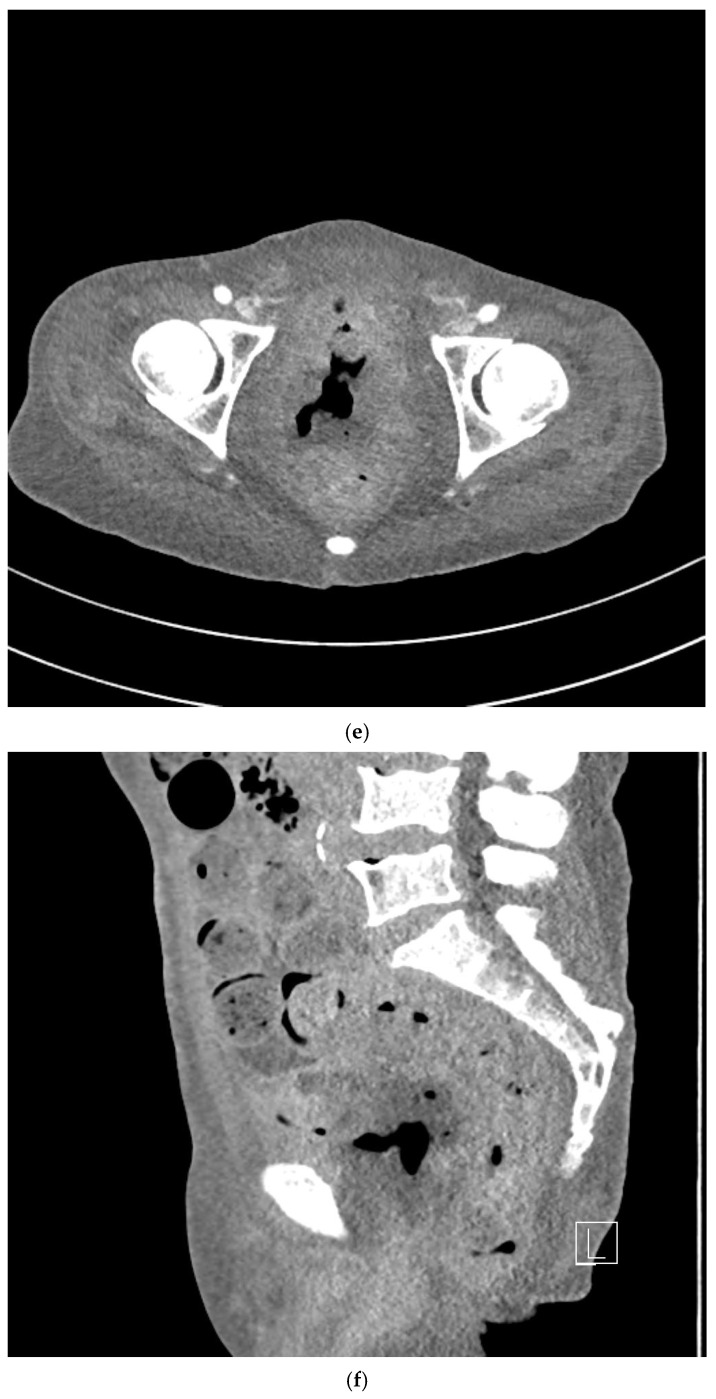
Serial computed tomography (CT) findings following definitive radiotherapy demonstrating tumor regression and subsequent necrosis with fistulous formation. (a,b) Contrast-enhanced CT images obtained one month after radiotherapy completion demonstrating a marked reduction in prostate tumor size, consistent with a significant treatment response. (c,d) Follow-up CT images obtained four months after radiotherapy completion showing extensive post-radiotherapy necrosis within the prostate and fistulous communication between the necrotic prostatic cavity and urinary bladder. (e,f) CT images obtained seven months after radiotherapy completion demonstrating persistent necrosis with maintained fistulous communication.
